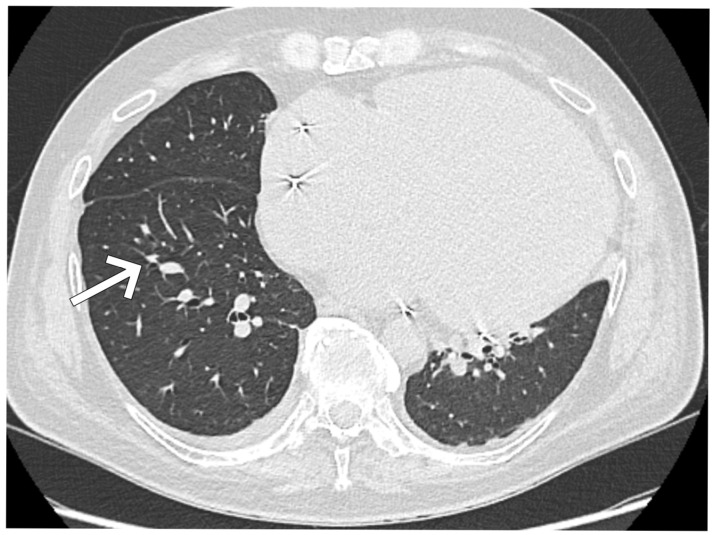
Chest CT (angio-CT) revealing multiple subpleural nodules up to 7 mm and enlarged mediastinal lymph nodes up to 13 mm. The white arrow indicates one of the representative subpleural nodules.
